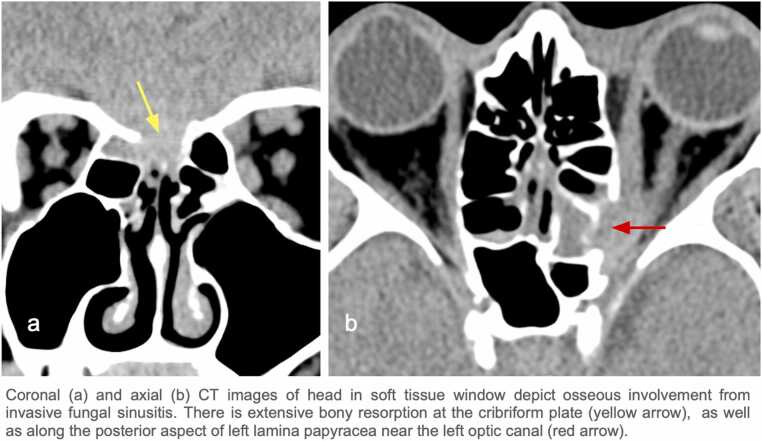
Coronal and axial CT images of head in soft tissue window depict osseous involvement from invasive fungal sinusitis.
